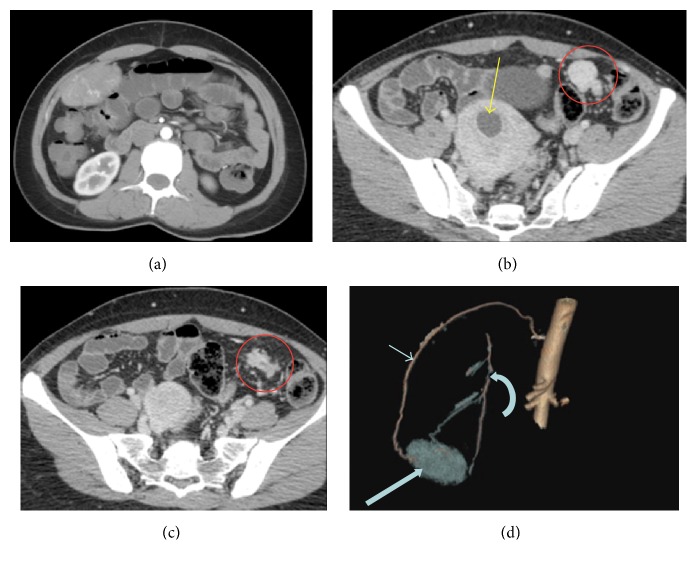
CT angiography. Axial section through right hypochondrium showed a well-defined oval shaped enhancing mass lesion along the peritoneal surface (a); axial section through the lower abdomen revealed few round well-defined homogenously enhancing soft tissue lesions in the mesentery (red circle in (b) and (c)) and yellow arrow shows a postsurgical changes in the uterus (b); volume rendering image of the right hypochondrium (d) shows the blood supply from right 10th intercoastal artery (thin arrow) and from the branch of right internal mammary artery (curved arrow). Thick arrow represents the mass lesion.
